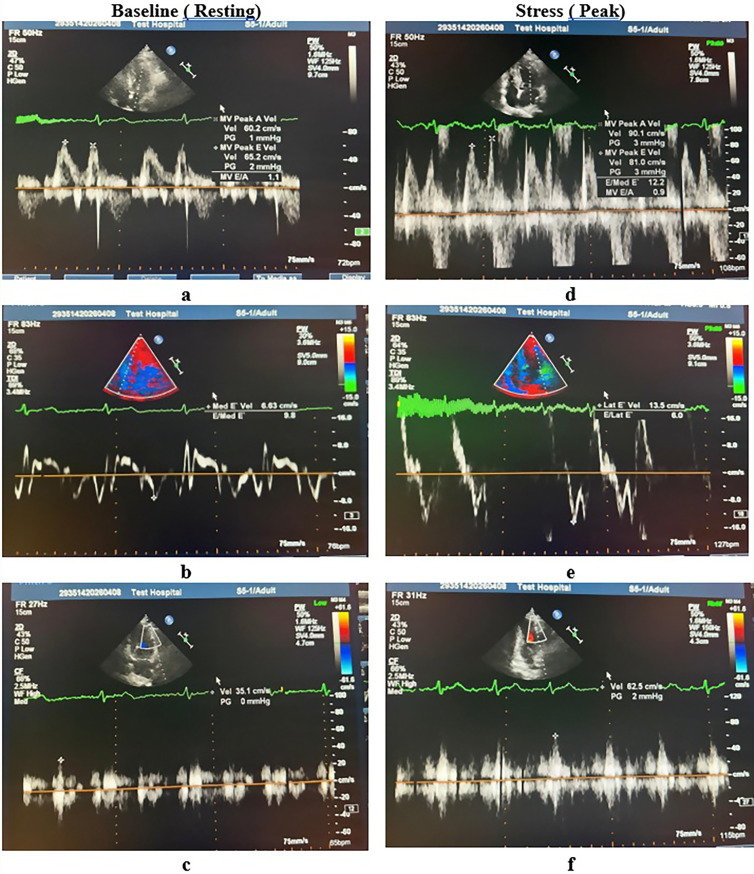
Dobutamine stress echocardiography during rest and stress. (a) Pulsed-wave Doppler of mitral inflow. At baseline, the E/A ratio is normal (>1.0). (b) TDI of the mitral annulus. Measurement of early diastolic myocardial velocity (e’) (baseline). (c) Pulsed-wave Doppler recording of the LAD coronary artery at the baseline stage. The distal LAD flow was identified in the anterior interventricular groove using colour Doppler guidance. The baseline peak diastolic velocity (bPDV) was measured at 35.1 cm/s. (d) Pulsed-wave Doppler recording of the mitral inflow at peak stress. (e) TDI of the mitral annulus during the peak stress stage. (f) Pulsed-wave Doppler recording of the distal LAD coronary artery during the hyperemic/peak stress stage. The Peak Diastolic Velocity (pPDV) was measured at 62.5 cm/s. LAD, left anterior descending; TDI, tissue Doppler imaging.
